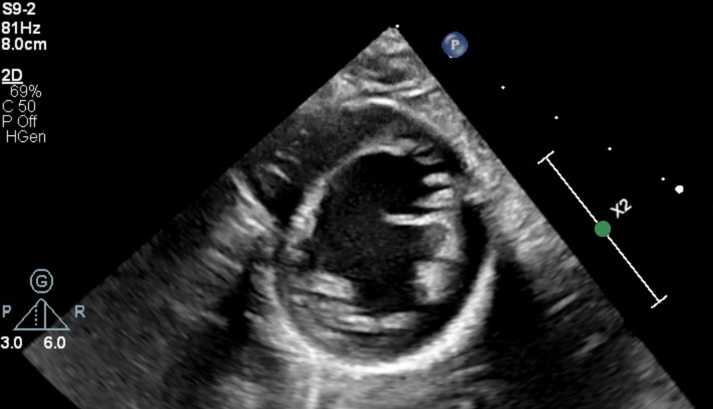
Hospital Follow-Up Echocardiogram Echocardiogram (parasternal short axis view) from first follow-up visit after hospitalization.
